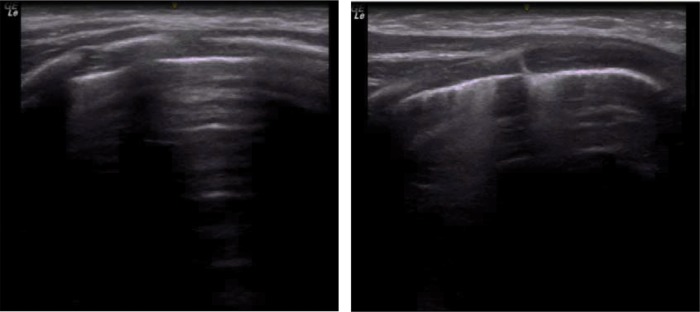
Chest ultrasound of the apex and lateral base of the right hemithorax. The image shows no consolidation and a pattern of interstitial involvement.
